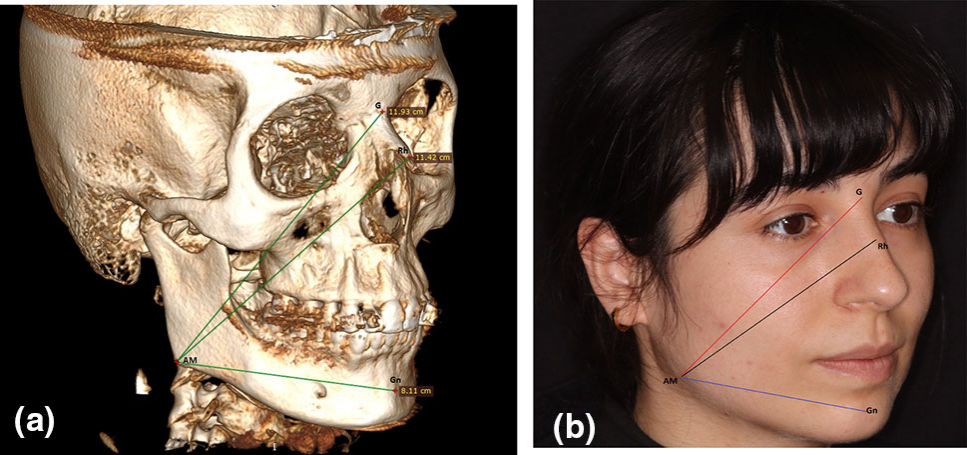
The measurement of distances between G-AM, Rh-AM, and Gn-AM on right side of the face were depicted through 3D CT (a) and photography (b). G: Glabella, Rh: Rhinion, AM: Angulus Mandibula, Gn: Gnathion
